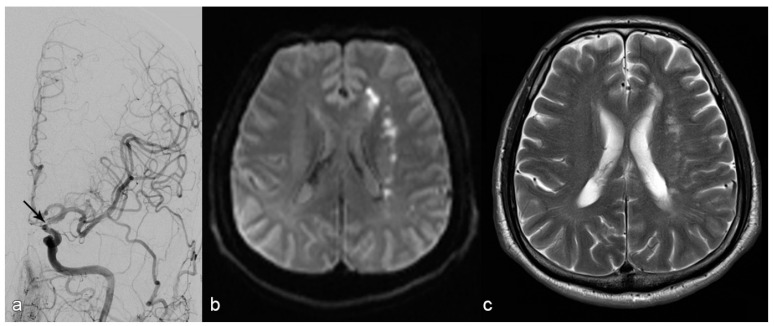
Patient with stroke due to stenosis in the terminal segment of the left internal carotid artery ((a): arrow). MR diffusion weighted imaging upon admission (b) shows subacute infarction in the white matter of the left centrum ovale with a hemodynamic pattern and without embolic cortical infarction. The decision was made to place a stent, which succeeded; MRI upon discharge showed no additional infractions (c).
